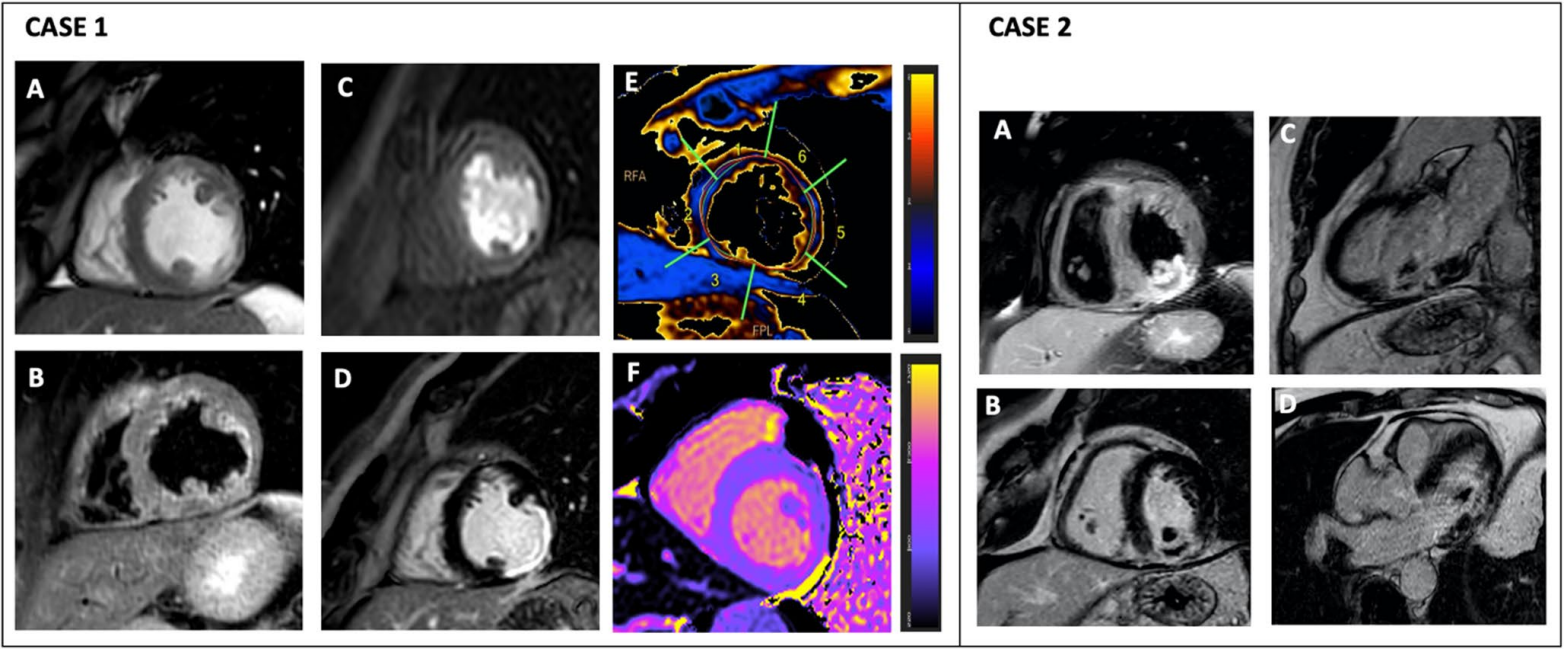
CMR evaluation in ACS.* Case 1*: CMR in a 38-year-old man post-AMI showed wall thinning in the mid-apical-lateral segment (1A), mild lateral wall edema on T2-weighted sequences (1B), perfusion deficit at rest (1C), and extensive subendocardial LGE (1D). Mapping revealed increased T2 (1E) and T1 (1F) relaxation times in the mid inferolateral segment. *Case 2:* CMR in a 75-year-old man after inferior STEMI showed edema in the mid-inferior segment, with hypointensity on T2 suggesting IMH (2A), transmural LGE confirming myocellular damage and ischemia affecting the postero-medial papillary muscle (2B, 2C, 2D). Abbreviations:AMI, acute myocardial infarction; STEMI, ST-elevation myocardial infarction; IMH, intramyocardial hemorrhage, and as inFigure 1
